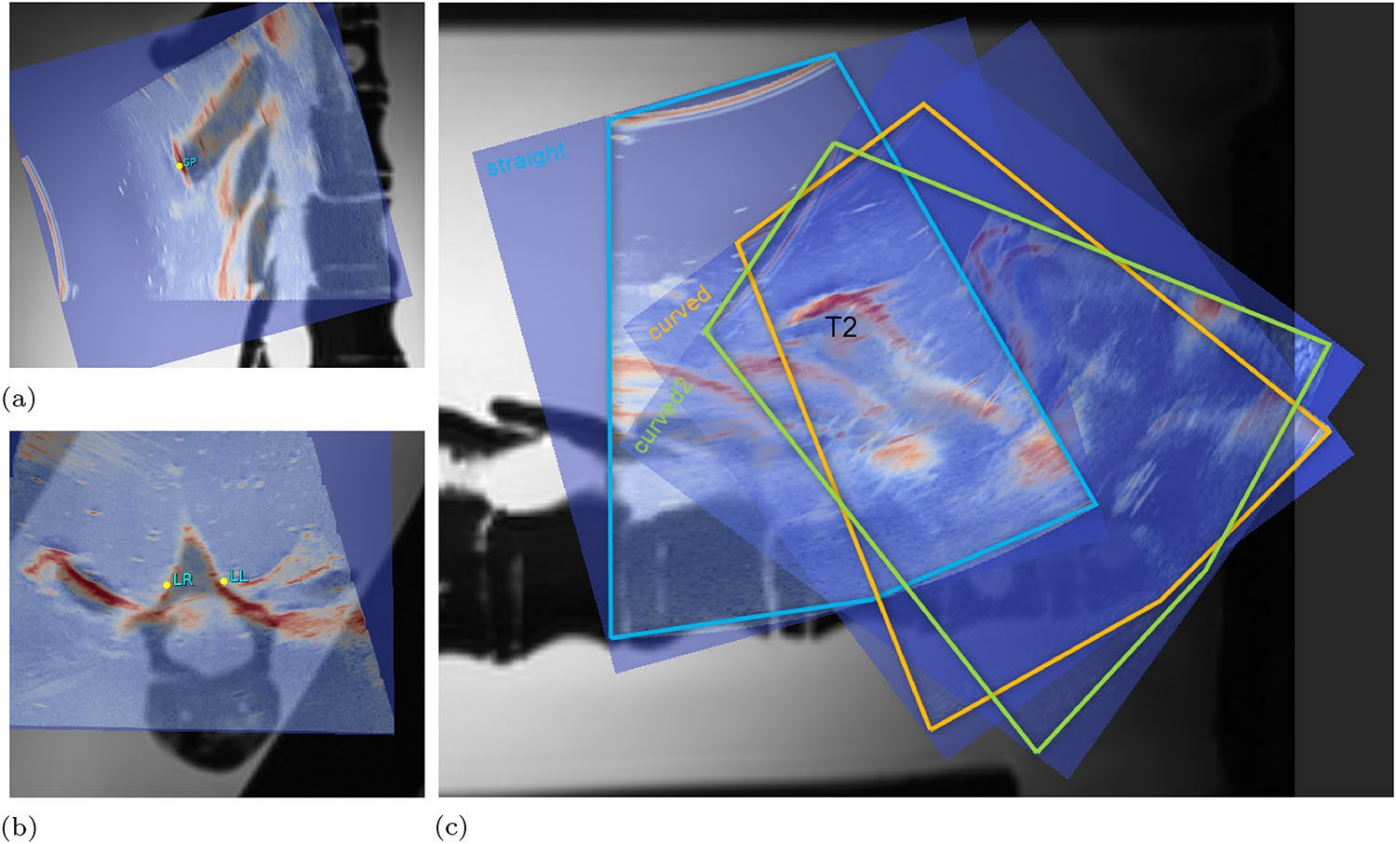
Landmark validation through manual US-MRI registration for the T2 vertebra in the sagittal plane in (a) and in the transverse plane in (b). Areas of strong US reflection are the spinous process (SPr) and the left (LL) and right lamina (LR), as indicated. In (c), all spine configurations $$\text {pos}_k$$ with $$k = 1, 2, 3$$ are visualised. The US-derived sensor tips $$S_{\text {pos}_k}^{\text {US}}$$ were selected individually for every US volume (straight: blue, curved 1: orange, curved 2: green) while US landmarks $$L_{\text {pos}_k}^{US}$$ were mapped from the straight configuration to the curved configurations
